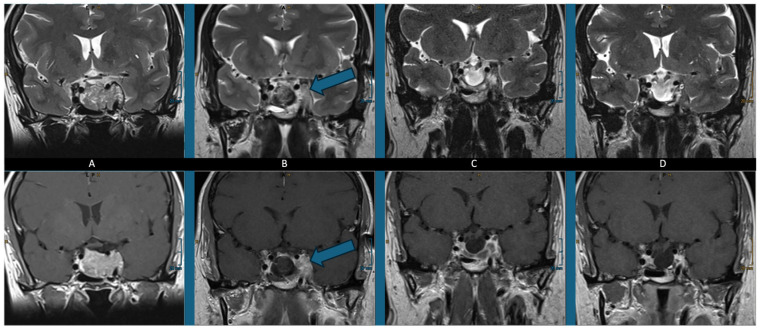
Demonstrative case of a 58-year-old woman with a nonfunctioning pituitary adenoma who presented with corticotropic insufficiency. Presented are T2 and contrast-enhanced T1 coronal sections of the sella. The preoperative MRI demonstrates a Knosp IV lesion with encased left ICA (A). The intraoperative MRI shows the resection cavity in the sella with a tumor remnant marked with the arrow (B). Further tumors could be removed after the intraoperative MRI leading to a gross total resection with no obvious remnant in the MRI after 3 months (C) and 1 year (D). No adjuvant treatment was administered.
